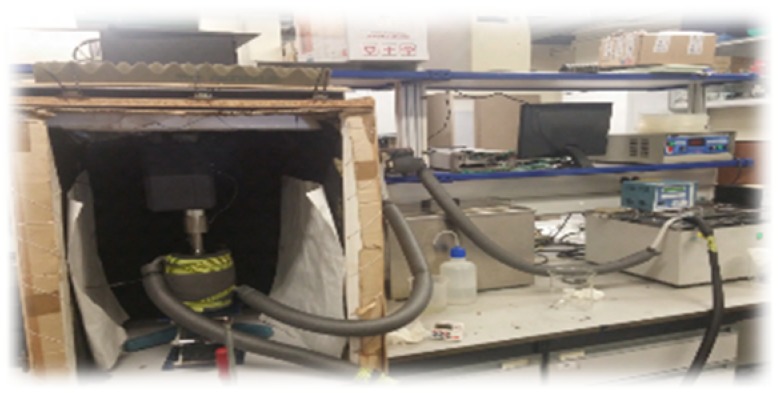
Experimental set-up for ultrasound assisted extraction.
